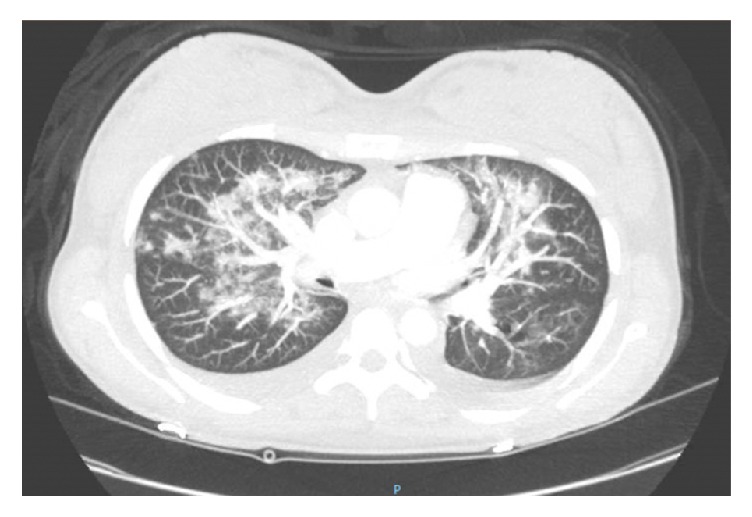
Repeat chest computed tomography angiography (CTA) on hospital day 5 featuring progressive ground-glass opacities bilaterally, which, in the setting of continued hemoptysis, was concerning for diffuse alveolar hemorrhage.
